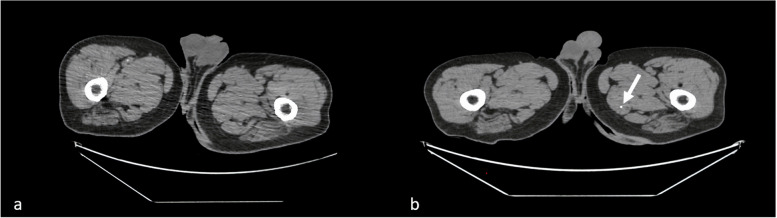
Noncontrast CT of the pelvis before (a) and after (b) prostatic embolization. Legend: Postembolization CT showing a punctiform NTE in the left pectineus muscle (white arrow)
